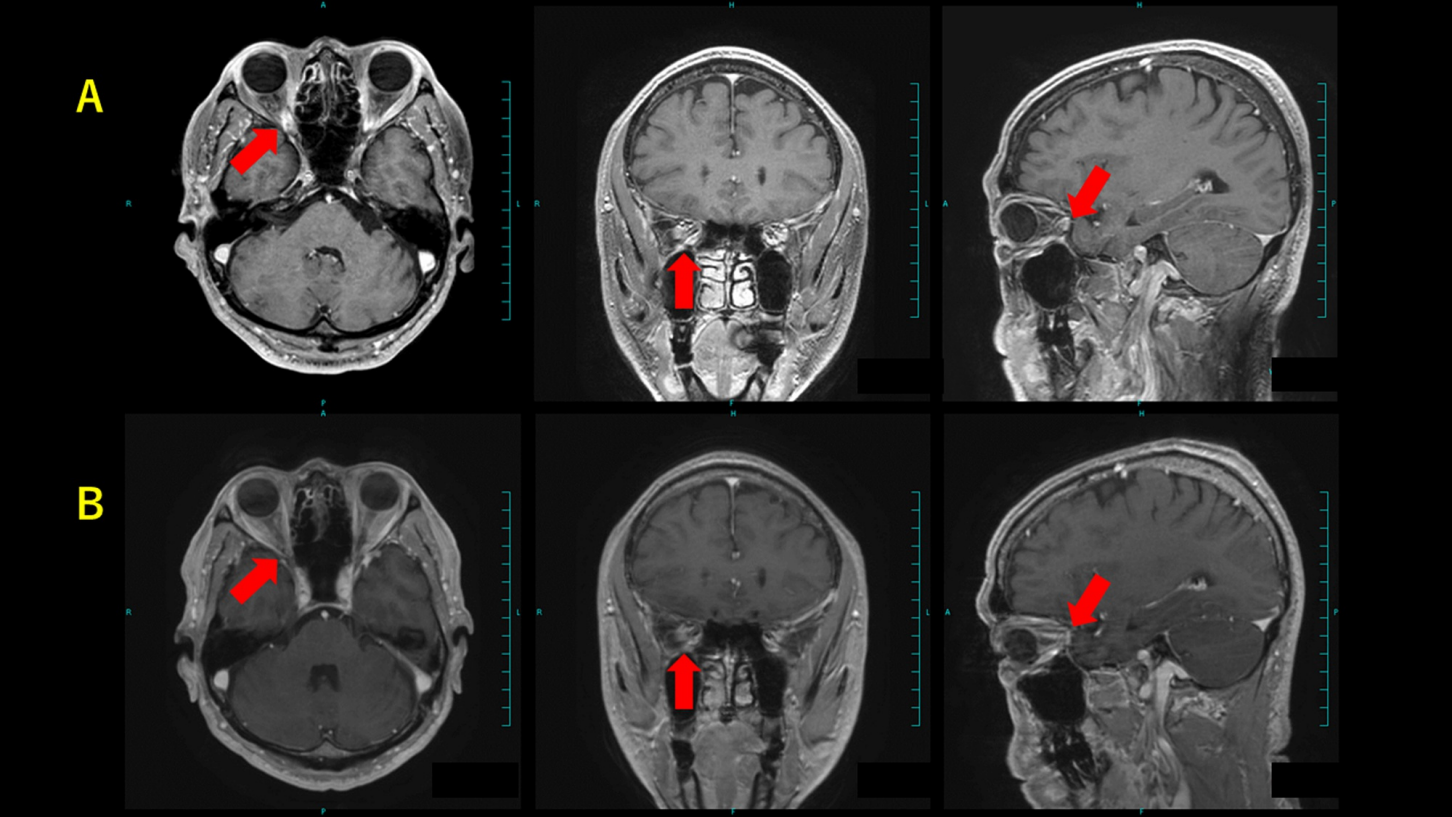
Brain MRI of case 2 Case 2 had decreased visual acuity and visual field narrowing due to the intra-optic canal tumor. (A) Before treatment and (B) 72 months after treatment. Red arrows denote tumor. The tumor size decreased; visual acuity and visual field were recovered.
